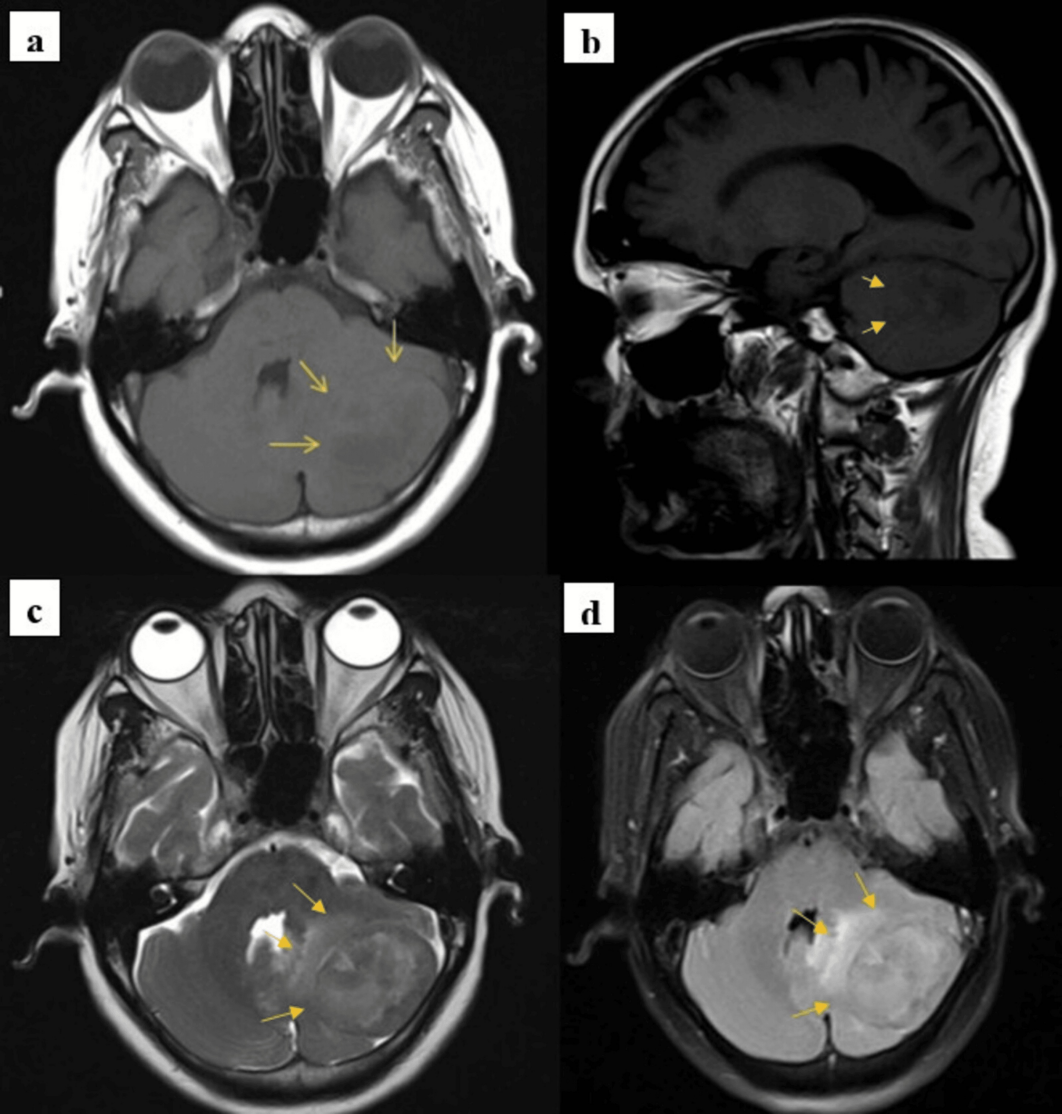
(a) MRI T1 axial section showing a large, well-defined, ovoid, extra-axial isointense to hypointense lesion in the left posterior fossa (yellow arrow). (b) MRI T1 sagittal section showing a large, well-defined, ovoid, extra-axial isointense to hypointense lesion in the left posterior fossa (yellow arrow). (c,d) T2/fluid-attenuated inversion recovery (FLAIR) images showing a hypointense-hyperintense lesion in the left posterior fossa with a broad base toward the dura (tentorium). The lesion is causing mass effect with compression of the adjacent cerebellar hemisphere, brainstem, and fourth ventricle.
